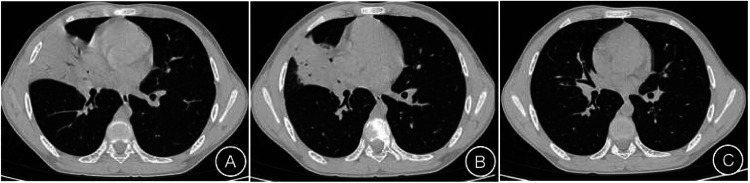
Chest CT imaging of a child with CPP presenting as lobar pneumonia. (12-year-old boy, admitted with cough for 5 days). (A) Initial CT shows extensive consolidation in the right middle lobe with relatively clear margins and visible air bronchograms. (B) Follow-up CT one week after bronchoscopy shows reduction in the extent of consolidation compared to the initial scan. (C) Follow-up CT one month after discharge (39 days after image A) demonstrates near-complete resolution of the consolidation.
